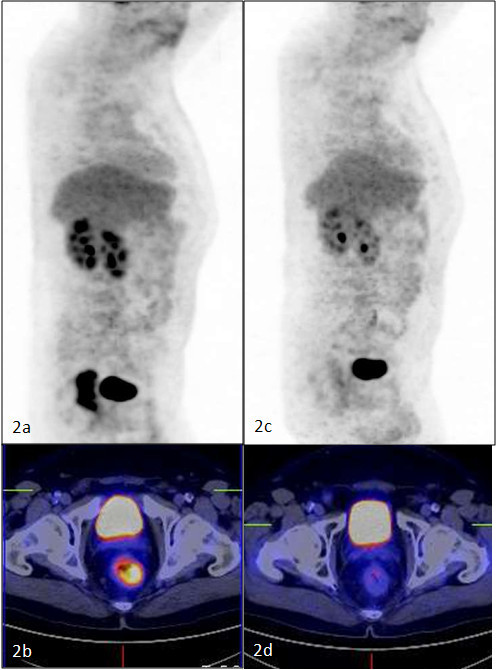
Complete metabolic response to CRT. 1A: Pre-CRT study - Intense rectal FDG uptake. 1B: Pre-CRT study - axial PET-CT images showing hypermetabolic rectal mass. 1C: Post-CRT study - Absence of rectal FDG uptake 1D: Axial PET-CT images showing rectal mass in CT images without FDG uptake.
